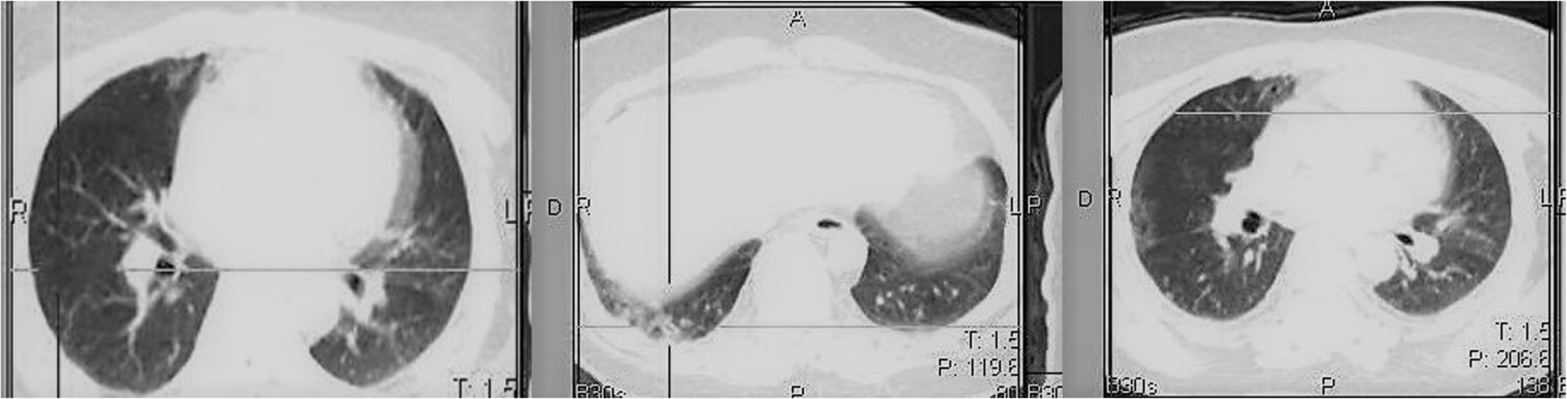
Axial CT images in different slices showing ground glass opacities in the right lung on the left and right images, with the middle image showing linear consolidation in the right lung. Note the predominant peripheral distribution
